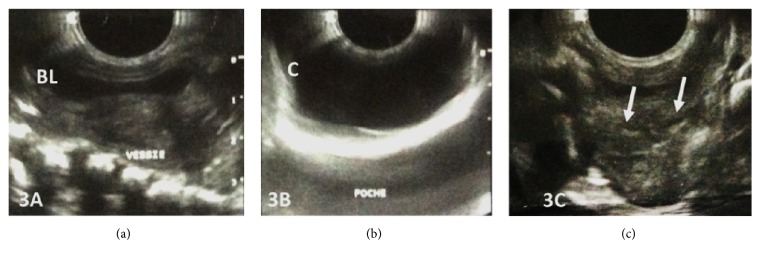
Postnatal pelvic and perineal ultrasound of the baby. (a-b) Suprapubic cross sections showing bladder (BL) and transperineal view showing cystic mass (C). (c) Uterus didelphys with the endometrial echogenic lines of the two hemiuteri (arrows).
